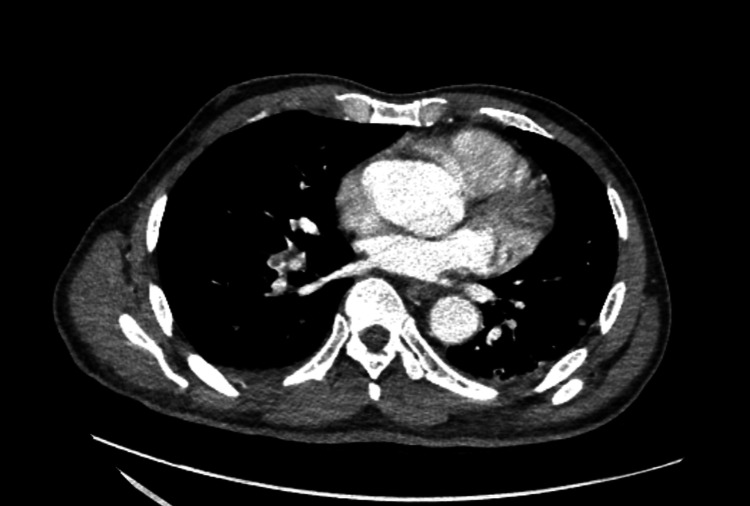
CT thorax showing bilateral mild-volume pulmonary emboli
